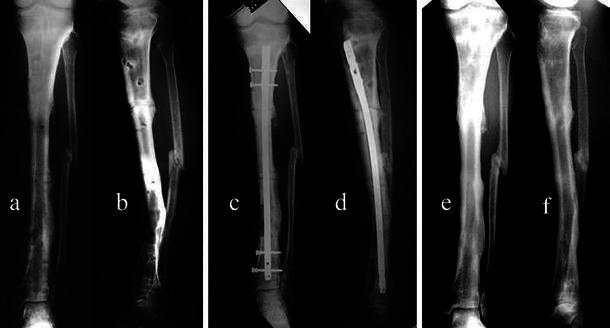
Case 5: a, b preoperative X-ray, 1 month after the removal of the fixator, c, d postoperative checkup, e, f follow-up 3 years later
